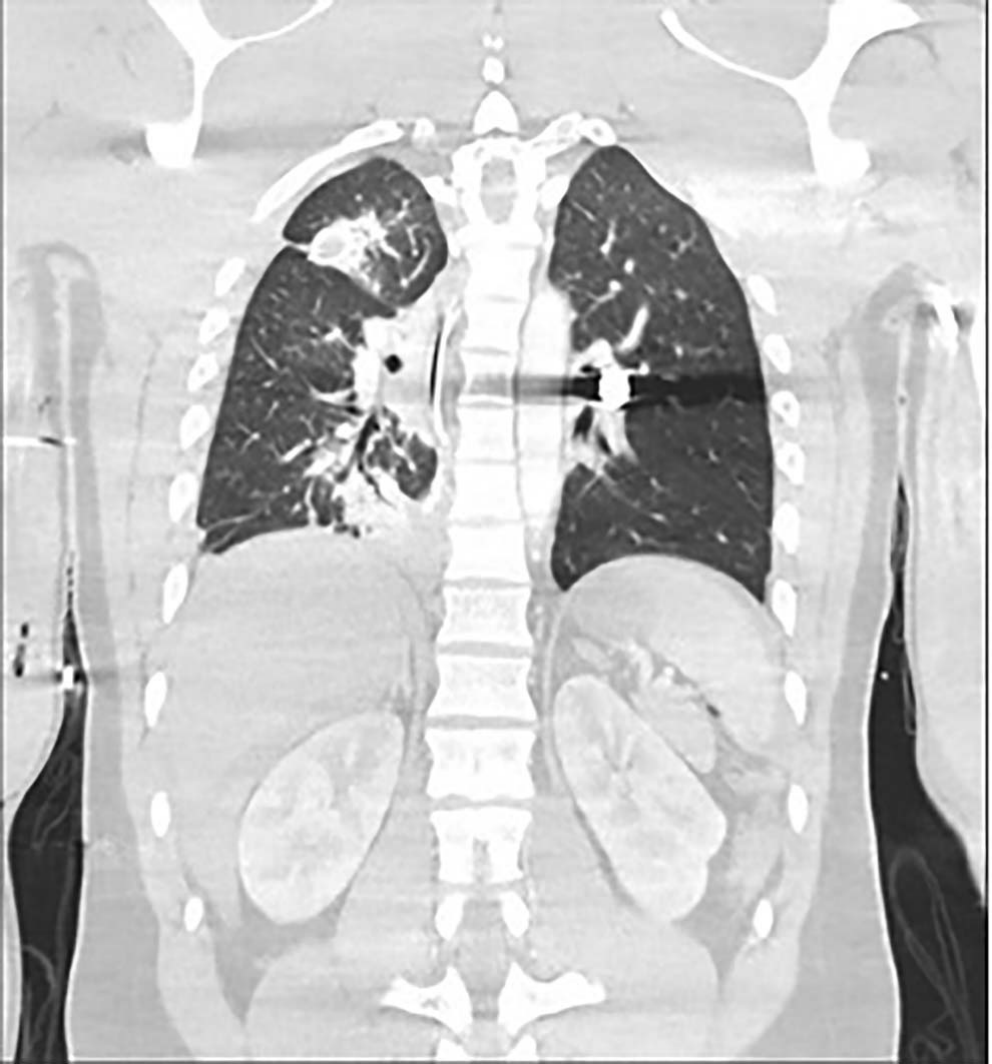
Preoperative computed tomography scan showed the bullet in the left pulmonary artery.
